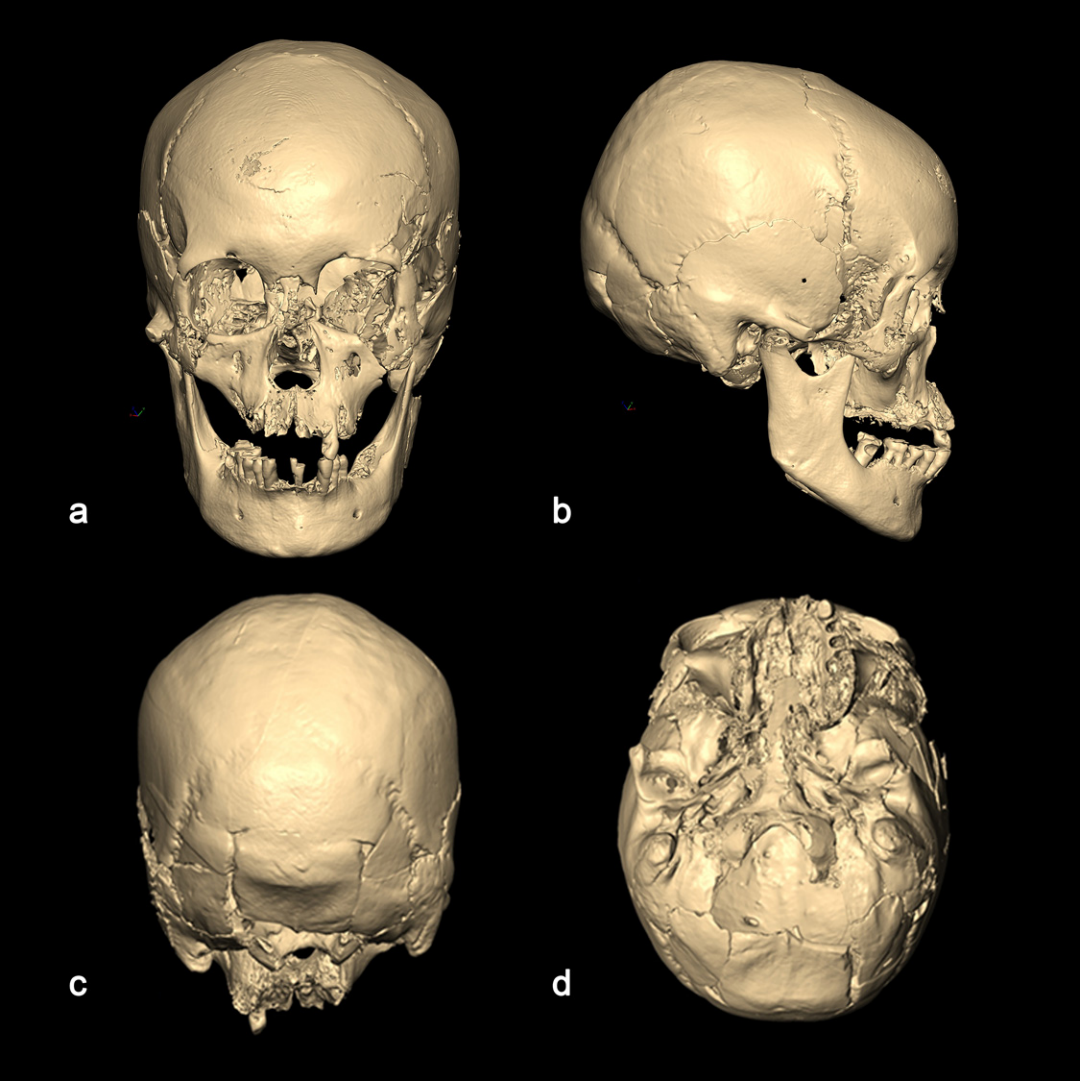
Reconstructed CT images of cranium (a, anterior view; b, lateral view; c, posterior view; d, basal view).
